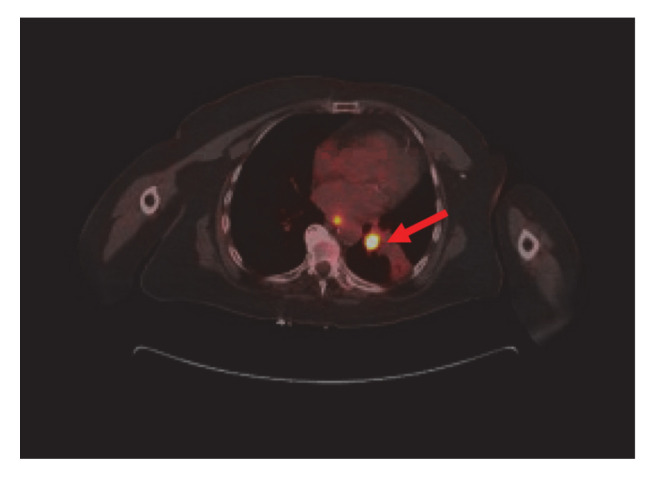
Nuclear Medicine Positron Emission Tomography/Computer Tomography (NM PET/CT): left lower lobe hypermetabolic neoplasm (red arrow) causing bronchial obstruction and distal collapse. Metastatic mediastinal adenopathy. Not shown, L3 vertebral body, left acetabulum pubis, and superior pubic ramus metastasis.
